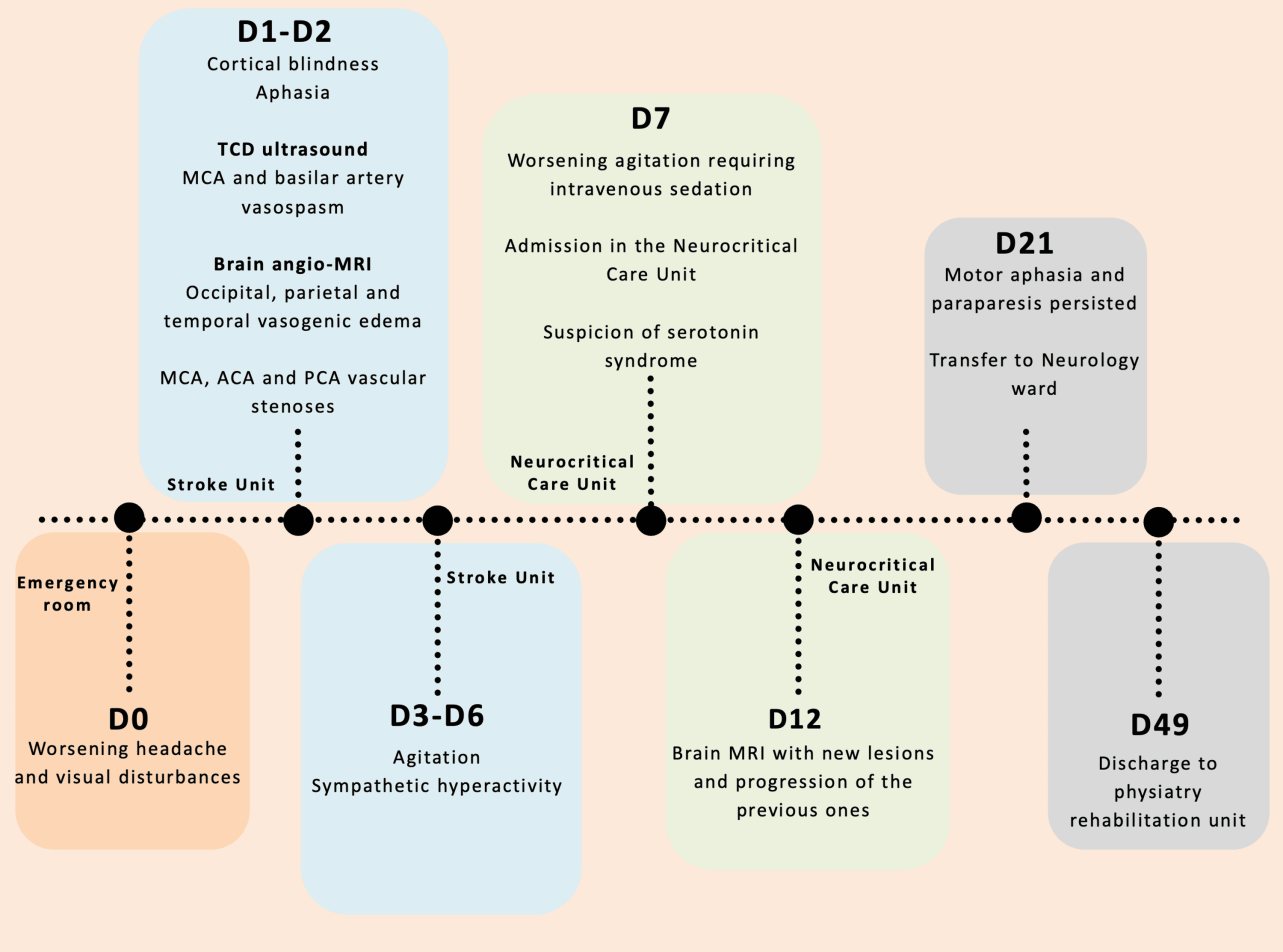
Chronological timeline summarizing key events ACA: anterior cerebral artery; MCA: middle cerebral artery; MRI: magnetic resonance imaging; PCA: posterior cerebral artery; TCD: transcranial Doppler
